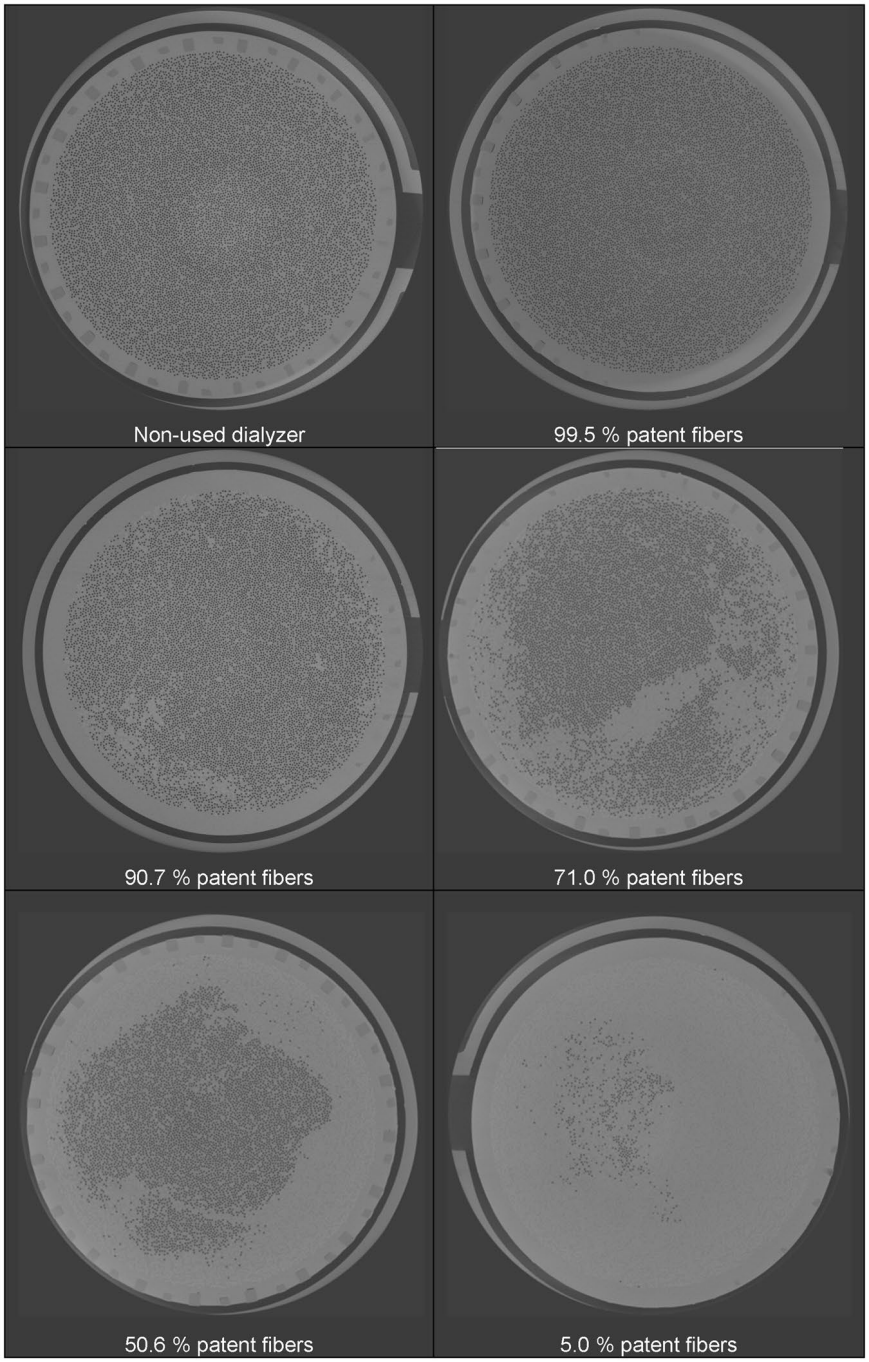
Representative CT images of dialyzer cross-sections (reference slice) showing different levels of coagulation.
